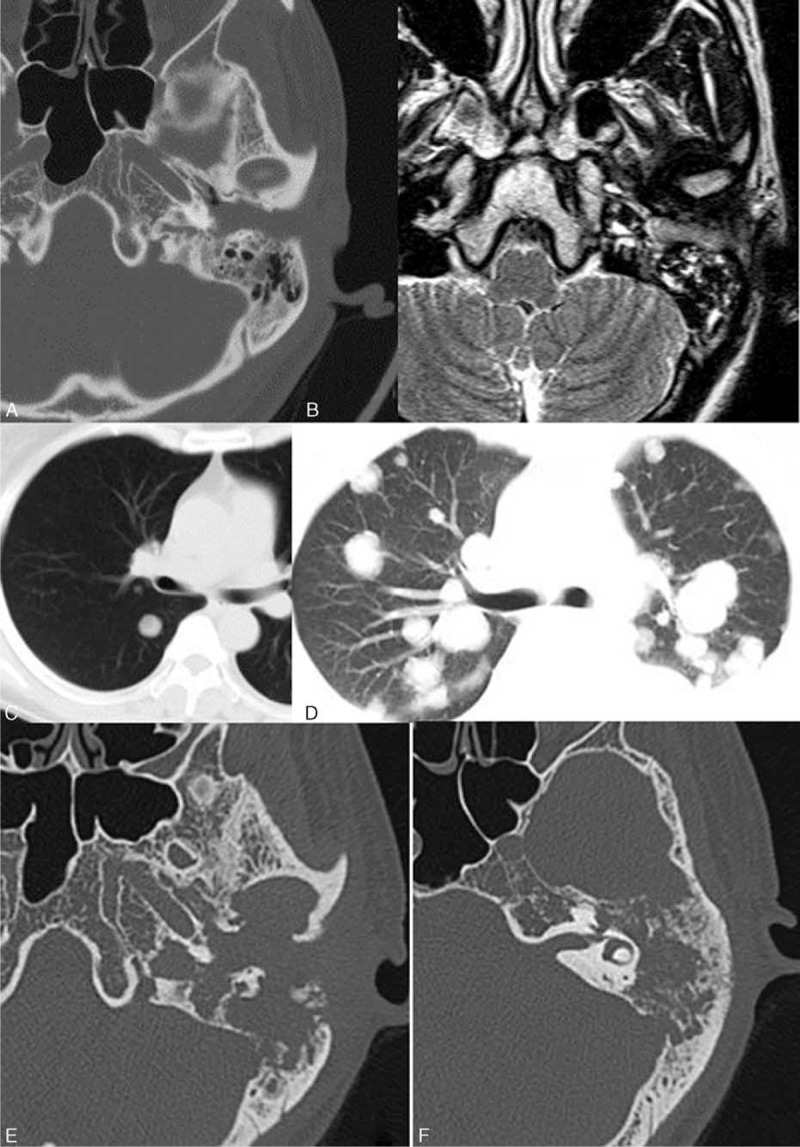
A 55-year-old female (case 25, cystic adenocarcinoma, stage 2) with complaints of mass in the left EAC for half a year with hearing loss for 4 months. HRCT and MRI are done. Axial HRCT reveals a soft tissue mass in the left EAC (A) with irregular destruction of the anterior and posterior wall of EAC. The lesion is iso-intense and locates in the left EAC as seen on T2WI (B). Multiple nodular metastases are seen on the lung CT (C). Operation was not done and only traditional medicine was given. Extensive metastases to the lungs (D) and temporal bone destruction are detected on the 28th month of follow-up (E). The patient died from lung metastases and local recurrence 28 months later. CT = computed tomography; EAC = external auditory canal; HRCT = high resolution computer tomography; MRI = magnetic resonance imaging.
